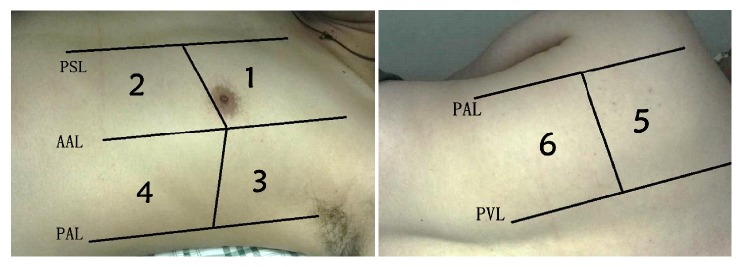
Regions of lung ultrasound examination. There are six examination regions on each side, delineated by parasternal line (PSL), anterior axillary line (AAL), posterior axillary line (PAL), and paravertebral line (PVL) [34, 35].
